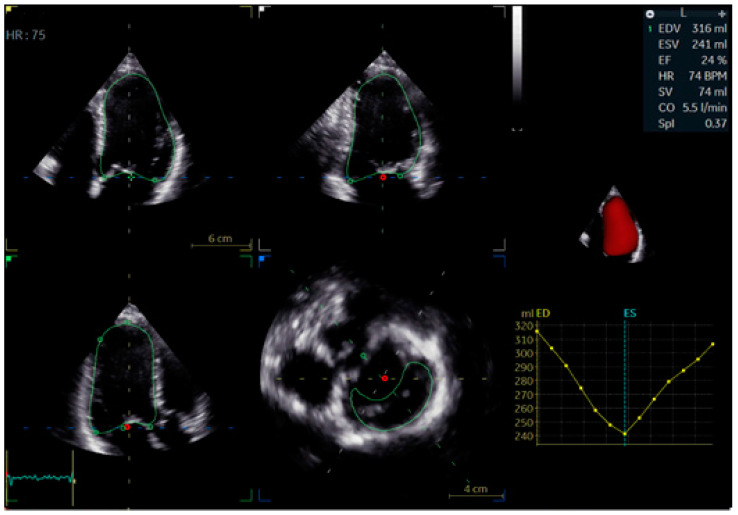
Three-dimensional, multi-view echocardiographic study in a patient with post-ischemic HFrEF.
